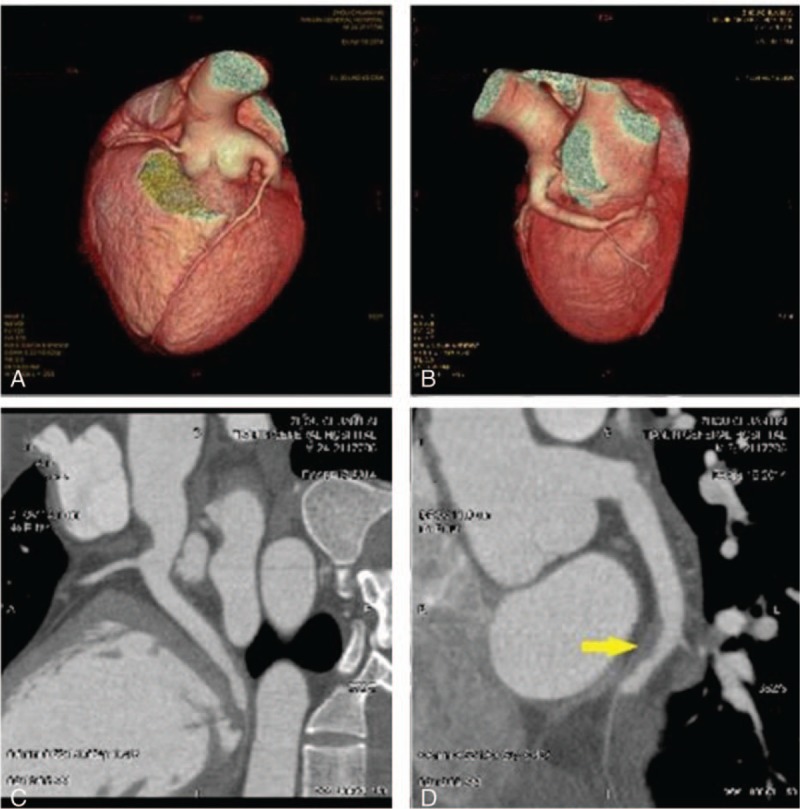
Sixty-four-slice coronary computed tomographic angiography images of the LCX 10 days after admission. A and B, Three-dimensional computed tomography reconstruction showing coronary artery ectasia involving the LM and LCX. C and D, A filling defect is shown in the distal portion of the LCX (arrow). LCX = left circumflex artery, LM = left main artery.
